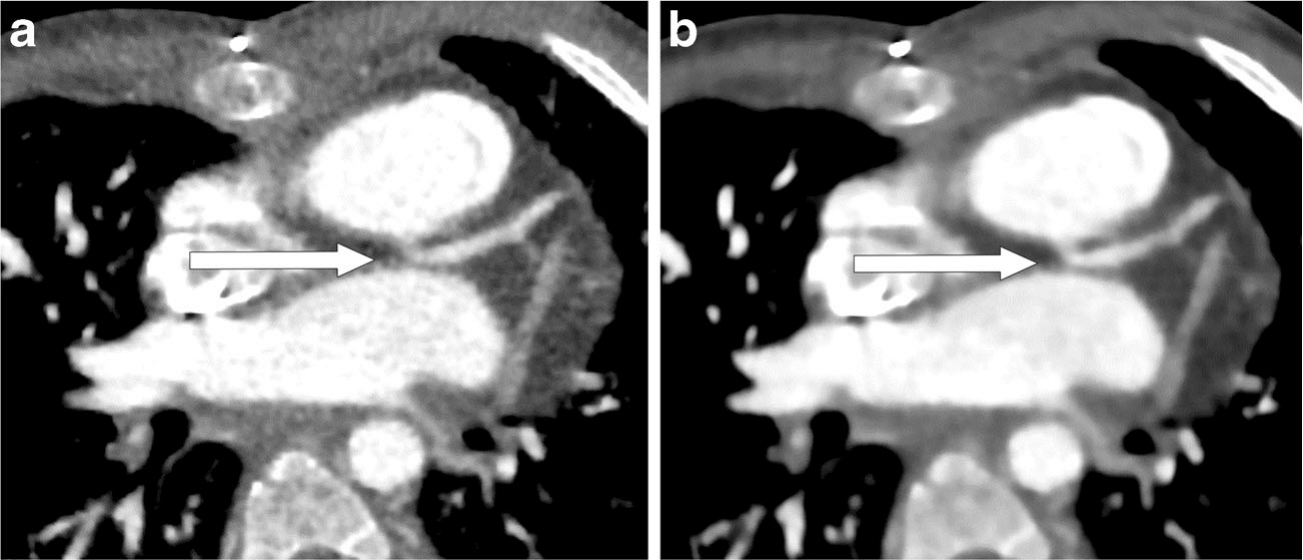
Axial contrast-enhanced CT images in a 5-year-old girl with truncus arteriosus. a Hybrid iterative reconstruction. b Full iterative reconstruction. The left main trunk originating from a noncoronary cusp (*arrow*) is demonstrated more clearly with full iterative reconstruction than with hybrid iterative reconstruction. The CT window settings of images in both figures are identical
